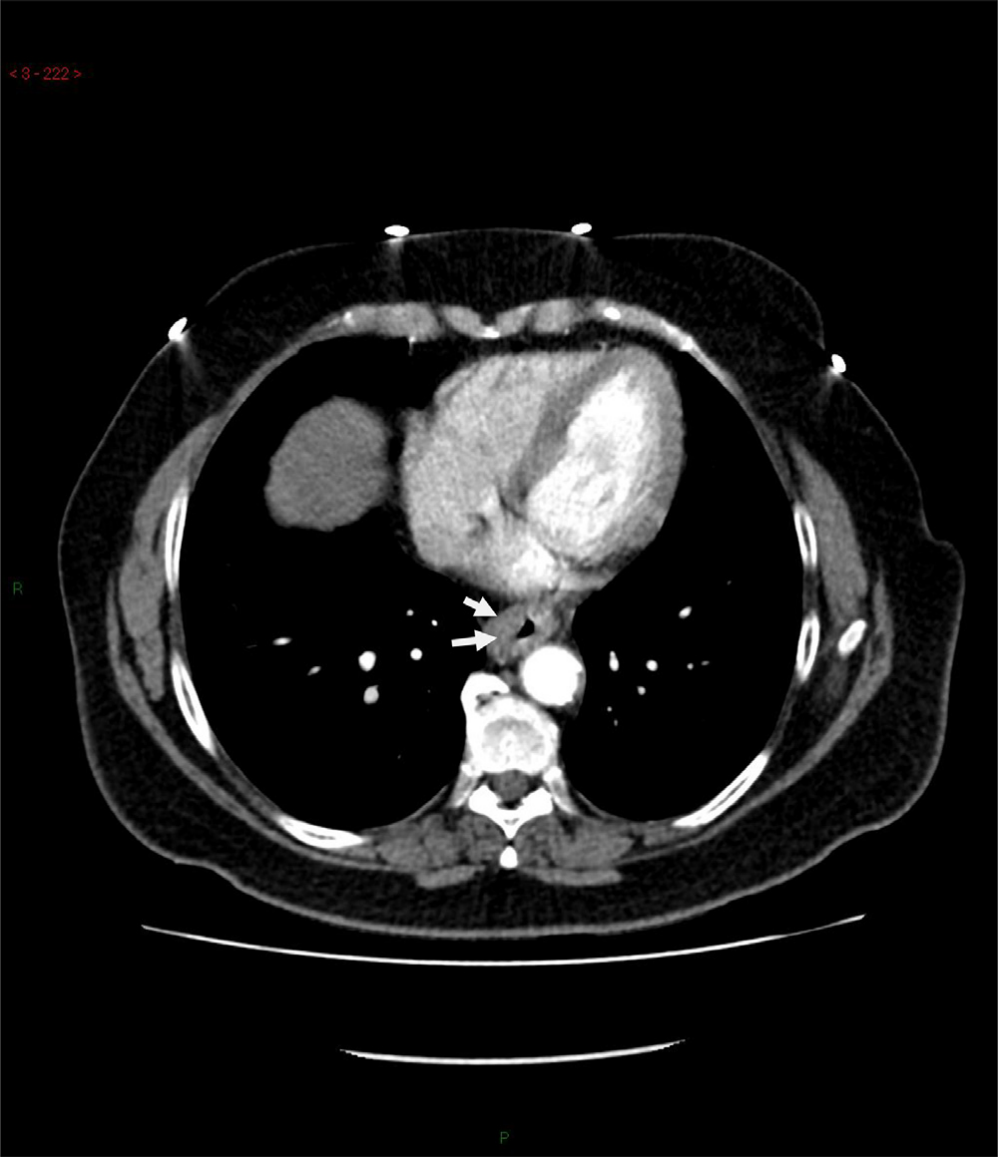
CT of the chest demonstrating thickening of the oesophagus (white arrows).
